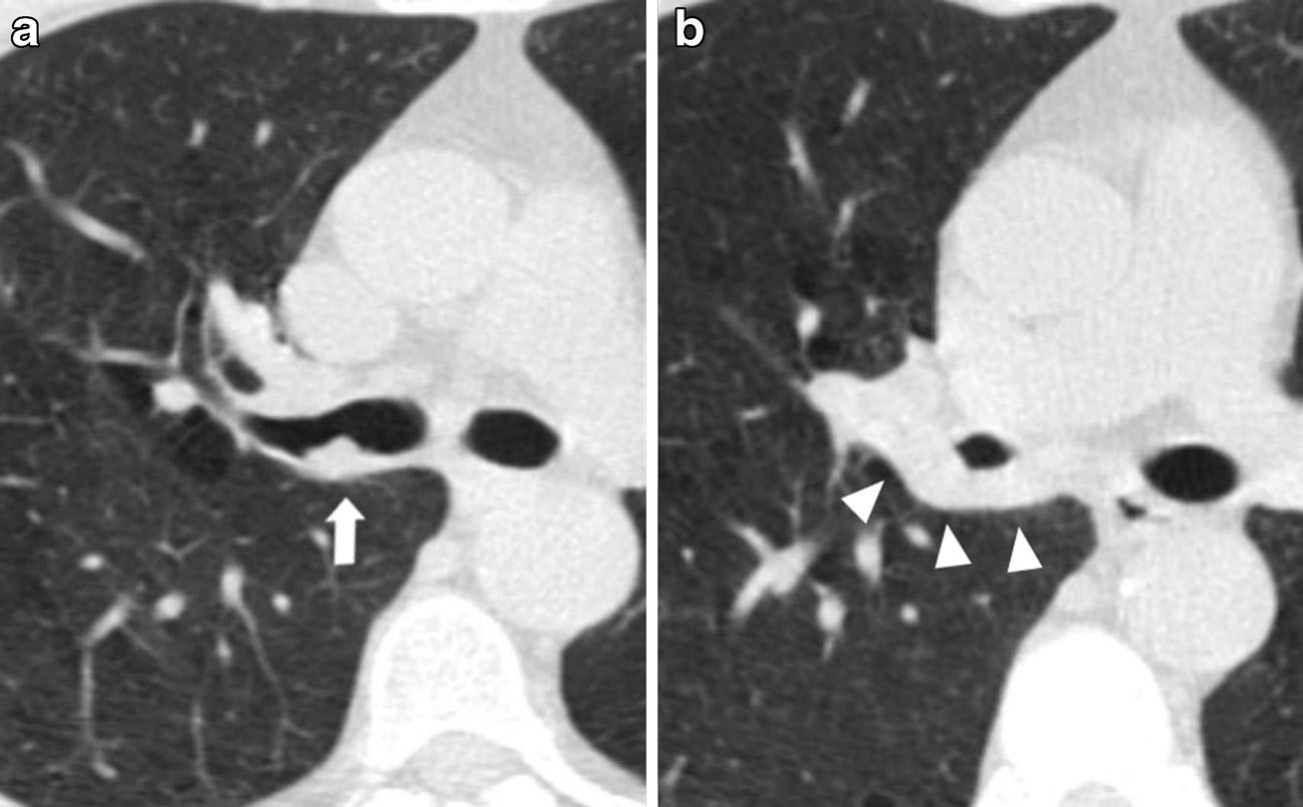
Endobronchial lung cancer. (a) Axial low-dose CT shows a small lesion abutting the posterior wall of the right main bronchus, interpreted as non-suspicious bronchial mucus (*arrow*). (b) Low-dose CT performed 1 year later shows a significant reduction of bronchial caliber due to the presence of neoplastic tissue (*arrowheads*)
